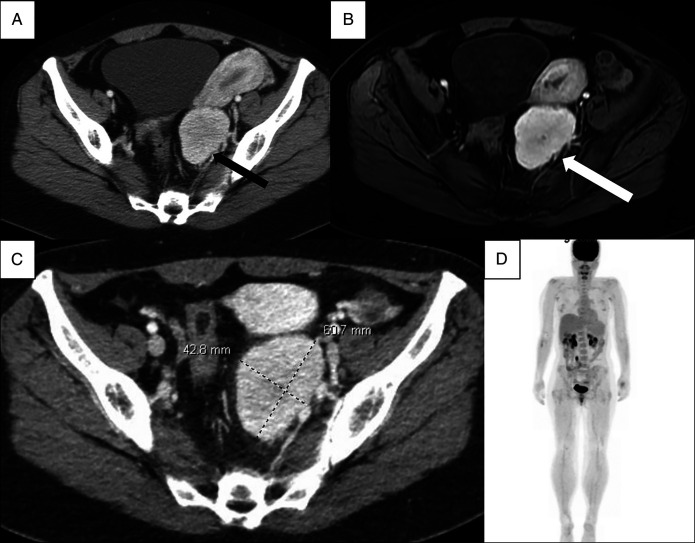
Radiographic imaging. Radiologically, the differential diagnosis included indolent lymphoma, paraganglioma, nerve sheath tumor, desmoid, or histoplasmosis recurrence. (A) Contrast-enhanced computed tomography, September 2019, (B) magnetic resonance of the abdomen—T1-weighted postcontrast, December 2022, (C) contrast-enhanced computed tomography, December 2023, (D) positron emission tomography scan, February 2024.
